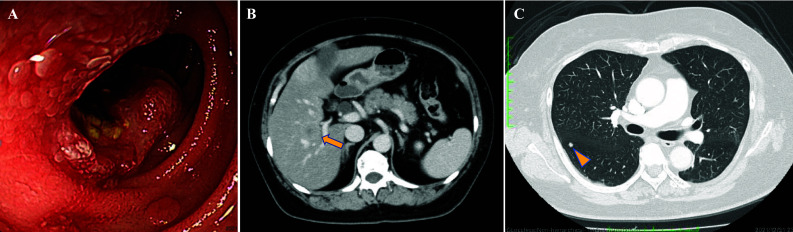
Endoscopic findings and computed tomography images at the time of diagnosis. A Endoscopic findings of the duodenum reveal a type 2 tumor on the descending part. B Abdominal computed tomography shows liver metastasis (long arrow). C Chest computed tomography reveals lung metastasis (arrow head)
